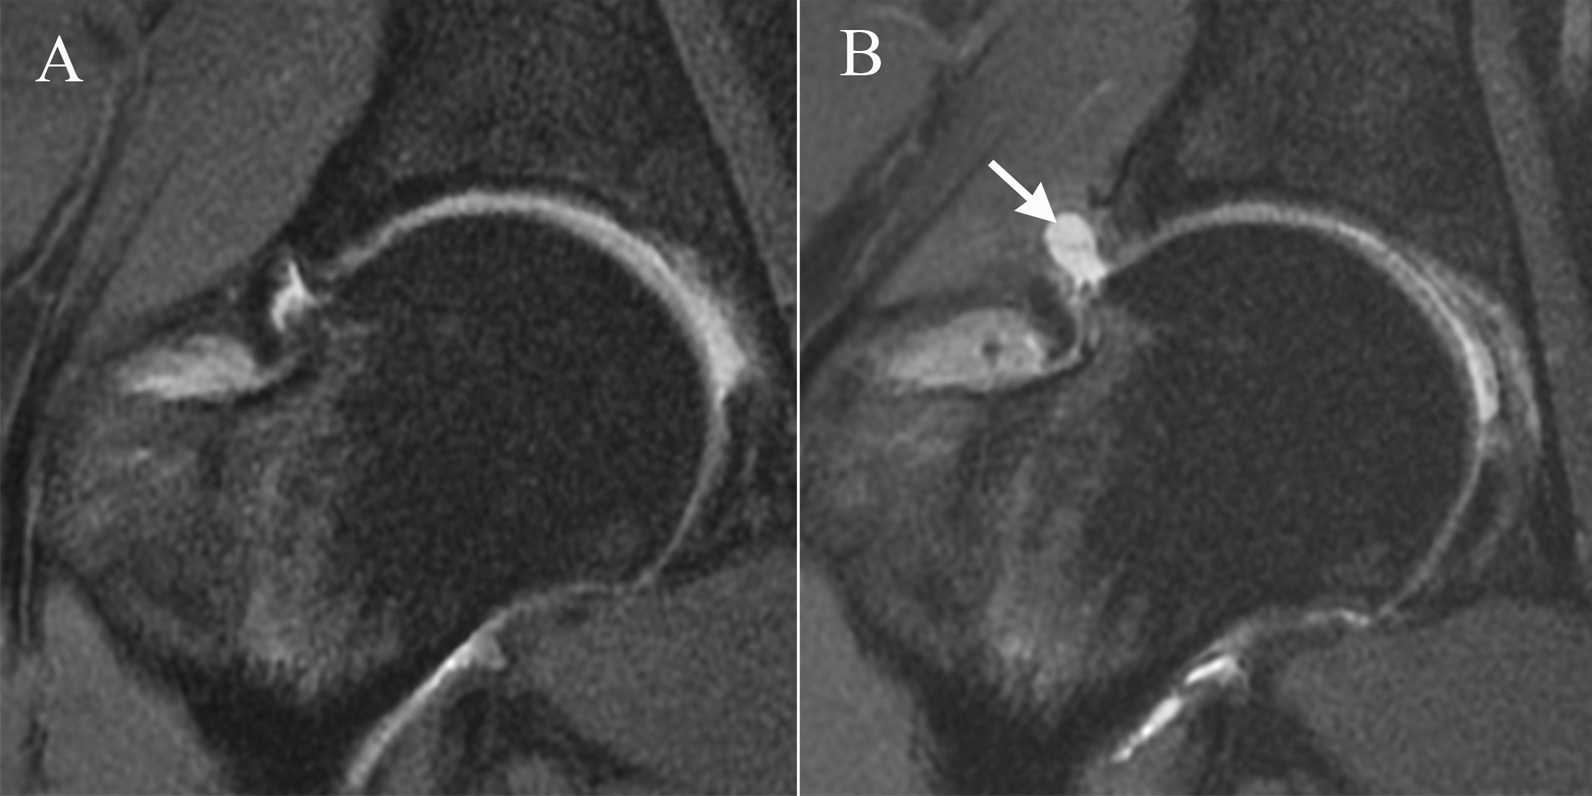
A Preoperative hip MRI showed continuous capsule. B MRI at 14 months after hip arthroscopy showed capsular defect (white arrow) in the same patient
